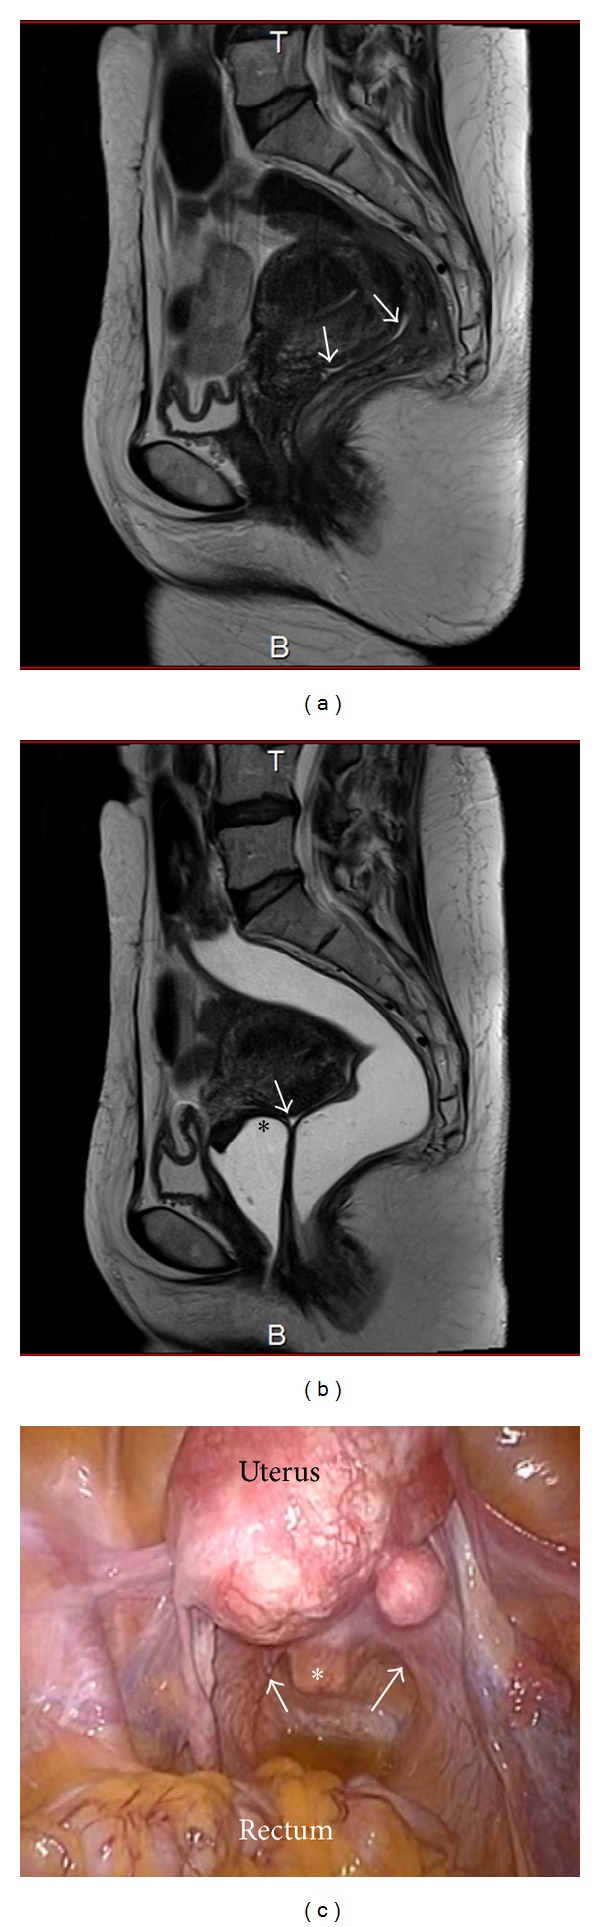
(a) MR image obtained before jelly administration in a patient with endometriosis but no CCDSO. Ascites is seen in the Pouch of Douglas (white arrow). (b) MR image obtained after jelly administration in the same patient. The surface of the rectal wall has a smooth appearance, and the posterior vaginal vault (asterisk) is visualized along with the ascites in the Pouch of Douglas (white arrow). (c) Laparoscopic findings in the same patient. The posterior vaginal vault is easily visualized in the area where the handle of the manipulator (asterisk) is seen. The uterosacral ligaments are also apparent (white arrow).
